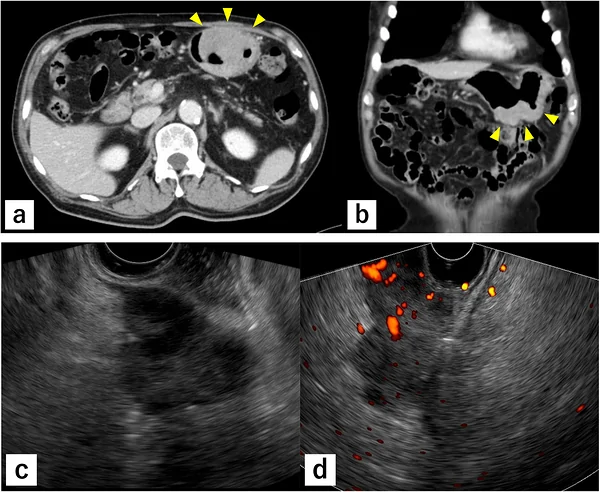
(a, b) Contrast‐enhanced computed tomography demonstrated a well‐enhanced, homogeneous gastric mass without significant lymphadenopathy or distant metastasis. (c) Endoscopic ultrasonography (EUS) revealed a 56‐mm lesion originating from the fourth layer of the gastric wall with heterogeneous echogenicity and partially anechoic areas. (d) Doppler imaging showed internal vascularity within the tumor.
